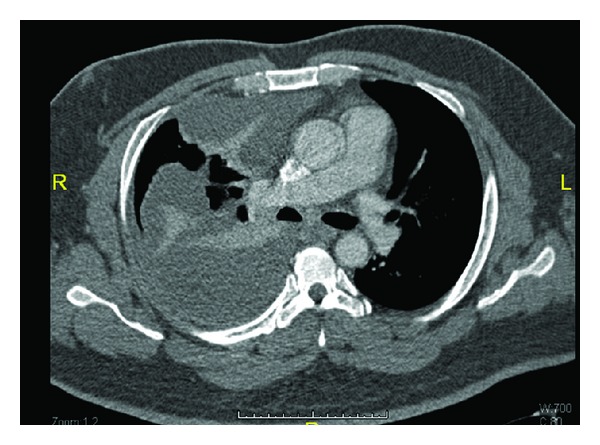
Chest CT showing the encysted pleural effusion.
